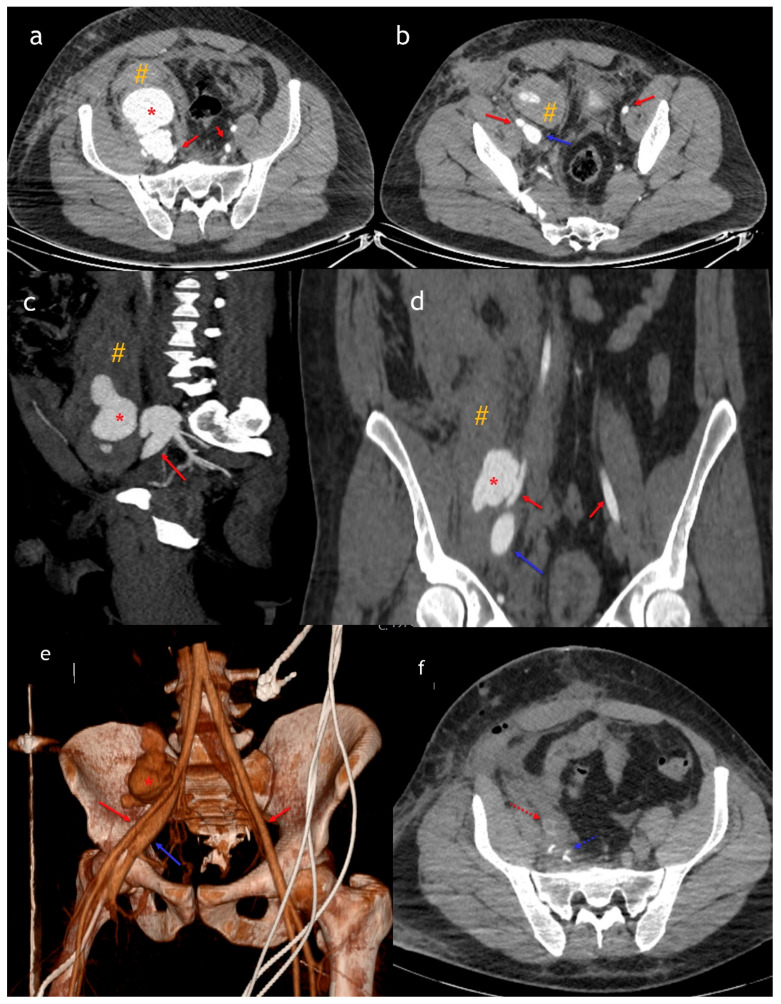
Axial (a,b), sagittal (c), coronal (d), and 3D (e) arterial phase CT images show a fistula and pseudoaneurysm [*] between the iliac artery [red arrow] and iliac vein [blue arrow], along with an accompanying hematoma. Postoperative imaging (f) reveals a stent placed in the iliac artery [red dashed arrow] and metallic clips at the level of the iliac vein [dashed blue arrow]. The patient underwent a second CT scan with arterial phase imaging a few hours later, which revealed a 6 cm diameter pseudoaneurysm connected to both the external iliac artery and vein. The inferior vena cava lumen demonstrated arterialization, while veins distal to the central venous catheter in the femoral vein showed normal enhancement. At our center, biphasic CTA [including both arterial and venous phases] is the preferred imaging modality when vascular injury is suspected. Abnormal communications between arteries and veins are defined as arteriovenous fistulas [AVFs] [5]. A second biphasic CTA confirmed an AVF between the right external iliac artery and vein, along with the arterialization of the vein. This phenomenon occurs due to the direct shunting of high-pressure arterial blood into the venous system, resulting in early and intense venous enhancement that mimics arterial opacification—a key imaging clue in the diagnosis of AVFs [6]. These can occur secondary to aneurysmal erosion or interventional procedures such as biopsies, surgery, or trauma. Compared to the craniocervical region, abdominal AVFs are relatively rare [1,5]. They can develop secondary to lumbar disk surgery or aneurysmal erosion—which may be associated with atherosclerosis, Ehlers–Danlos syndrome, Marfan syndrome, neoplastic invasion, or mycotic and syphilitic aneurysms—or penetrating trauma [6]. Trauma is a rare cause of AVF, with an incidence ranging from 2.3% to 3.9% [7,8]. Endovascular therapy is generally a less invasive and safer treatment option in hemodynamically stable patients, whereas open surgery remains the first-line treatment for unstable trauma patients, as was the case here [6]. The recent literature also highlights the importance of endovascular techniques in managing arteriovenous fistulas and related complications, as demonstrated by Ascoli Marchetti et al. in their case report on aortocaval fistula treatment [6]. Given the rarity of abdominal AVFs, hematomas, and pseudoaneurysms following gunshot wounds, accurate diagnosis and prompt treatment are essential for survival. This study aims to highlight the diagnostic challenges and treatment strategies in managing such abdominal vascular injuries.
